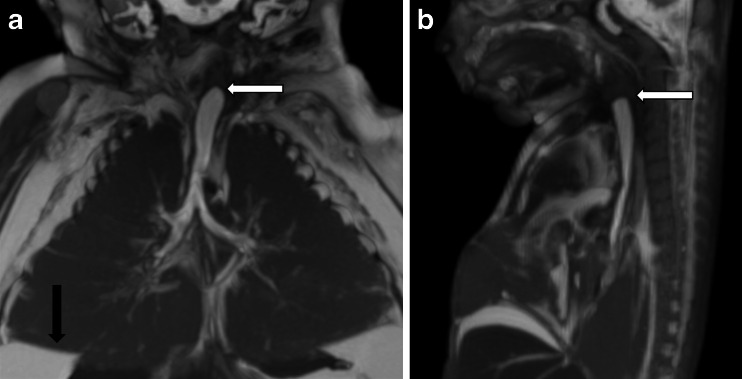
Case 1. Postmortem MRI in a male foetus, same case as in Figs. 1 and 2, with congenital high airway obstruction syndrome. Coronal (a) and sagittal (b) planar reconstructions show the dilated airway below the level of the obstruction. Note the level of the obstruction with distal fluid-filled airway (*white arrow*) and the eventration of the diaphragm (*black arrow*)
